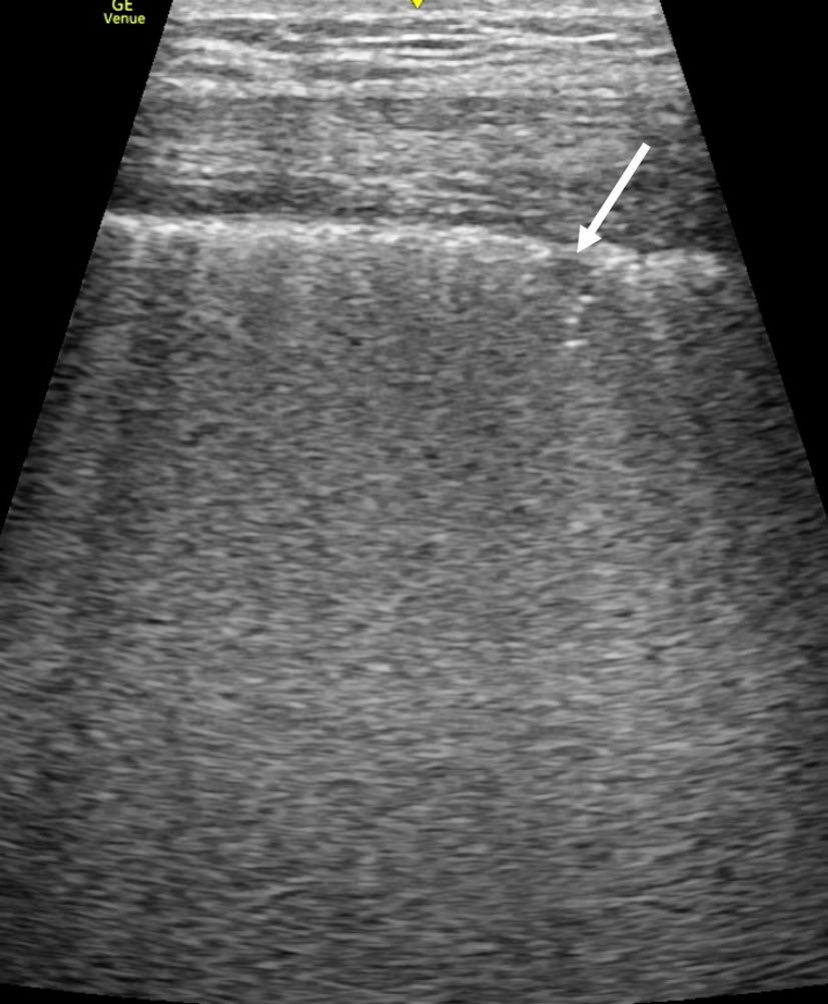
A lung ultrasound image of COVID-19 pneumonia showing coalescent B-lines with a small subpleural consolidation (arrow)
